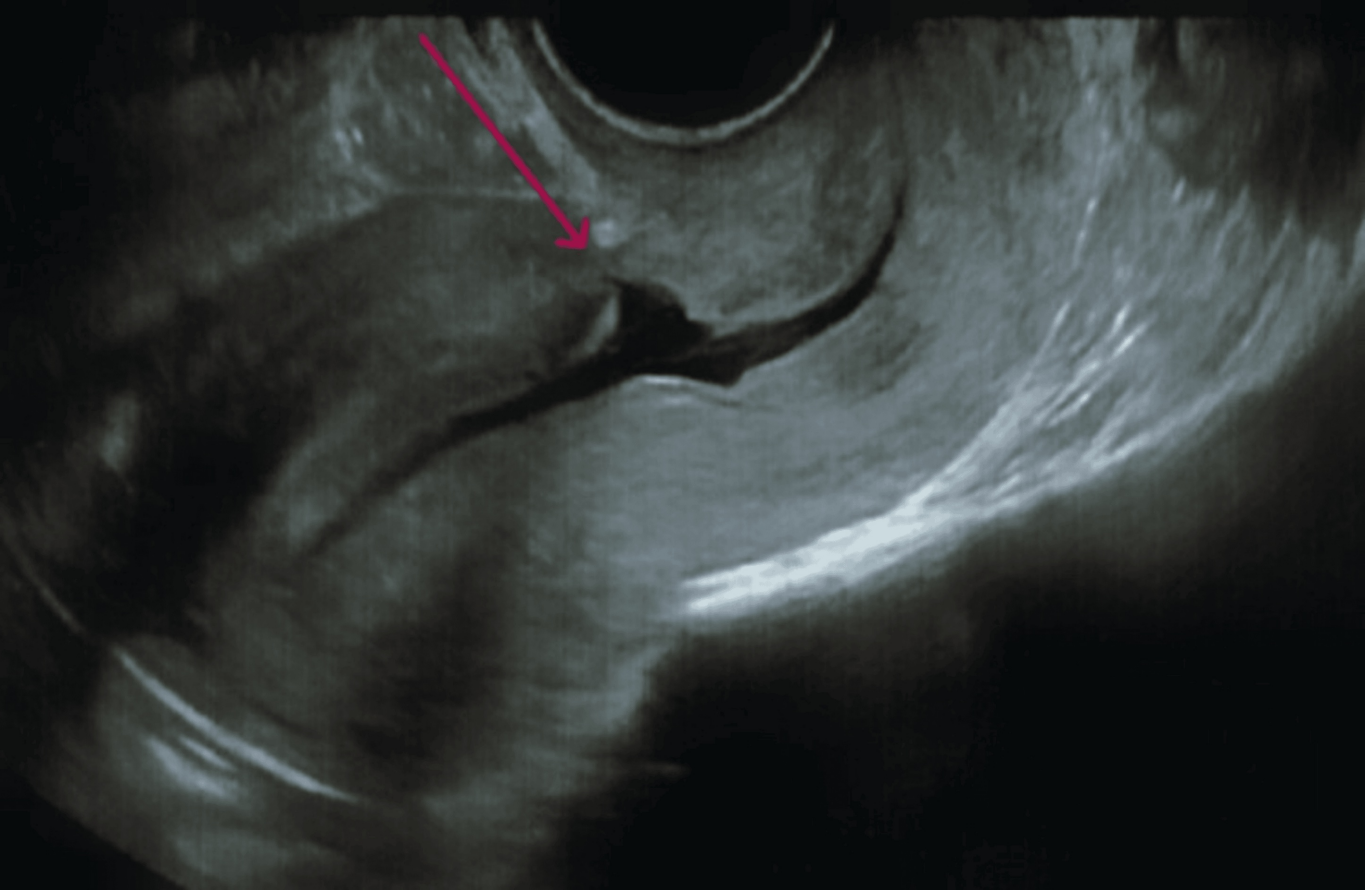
Ultrasonography showed a V-shaped defect noted at the site of the prior caesarean scar, measuring 24 x 6 x 6 mm, with a myometrial thickness of 2 mm, with the base at the endometrial cavity and apex towards the anterior wall of the isthmus, suggestive of an isthmocele.
